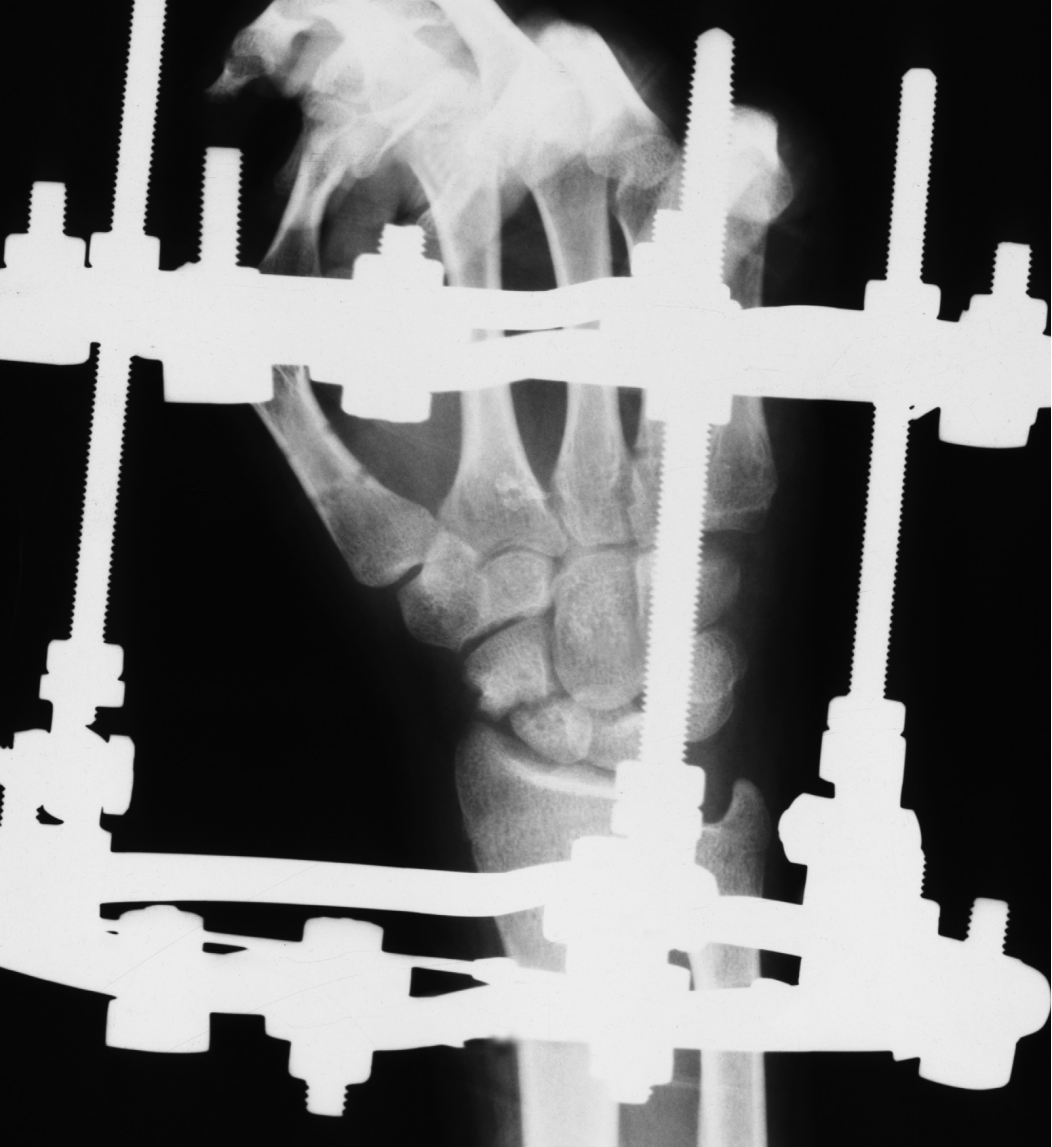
A radiograph of the SNU in patient number 3 with the frame in situ.
